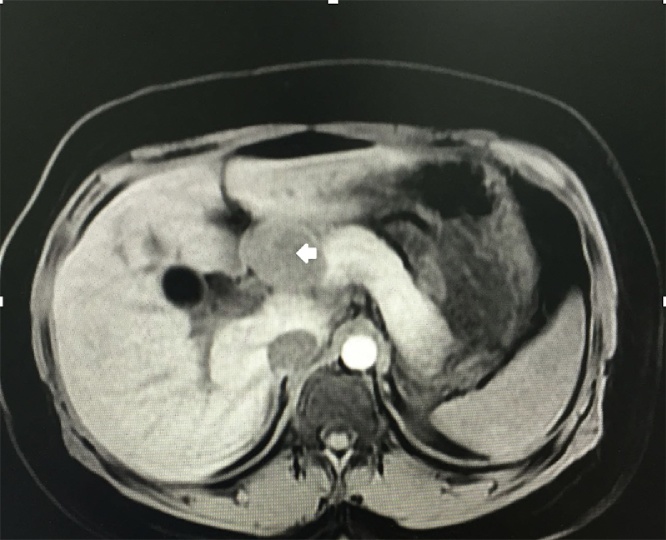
MRI axial T1 without contrast. The arrow points to the isointense 4 cm marginated mass in the pancreas head.
